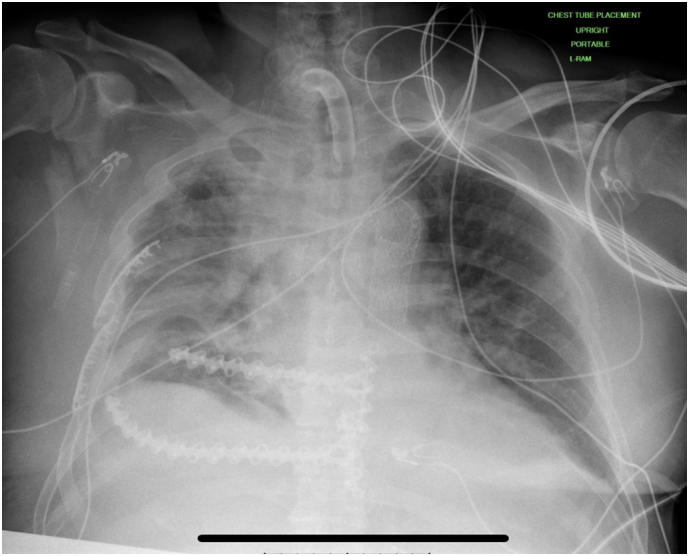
Chest X-ray demonstrating the surgical stabilization of rib fractures, once again showing the offset placement along the sternum. Noted is the stent placement from the thoracic endovascular aortic repair and the tracheostomy.
